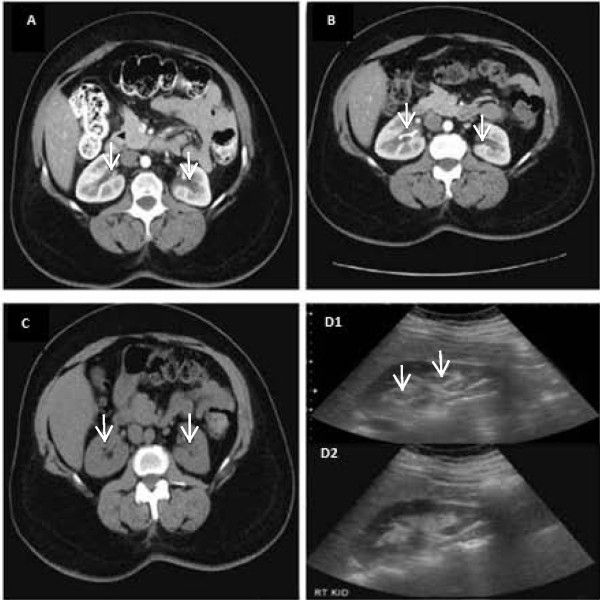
Time course of hydronephrosis. (A) Abdominal computed tomography. Initial presentation; mild to moderate bilateral hydronephrosis. (B) Abdominal computed tomography. Persistent hydronephrosis despite ureteral stent placement in right kidney. (C) Abdominal computed tomography. Persistent hydronephrosis after exploratory laparoscopy and right oophorectomy. (D) Ultrasound of right kidney. (D1) Mild hydronephrosis prior to reduction of fluid intake. (D2) Resolution of hydronephrosis after fluid intake reduction.
